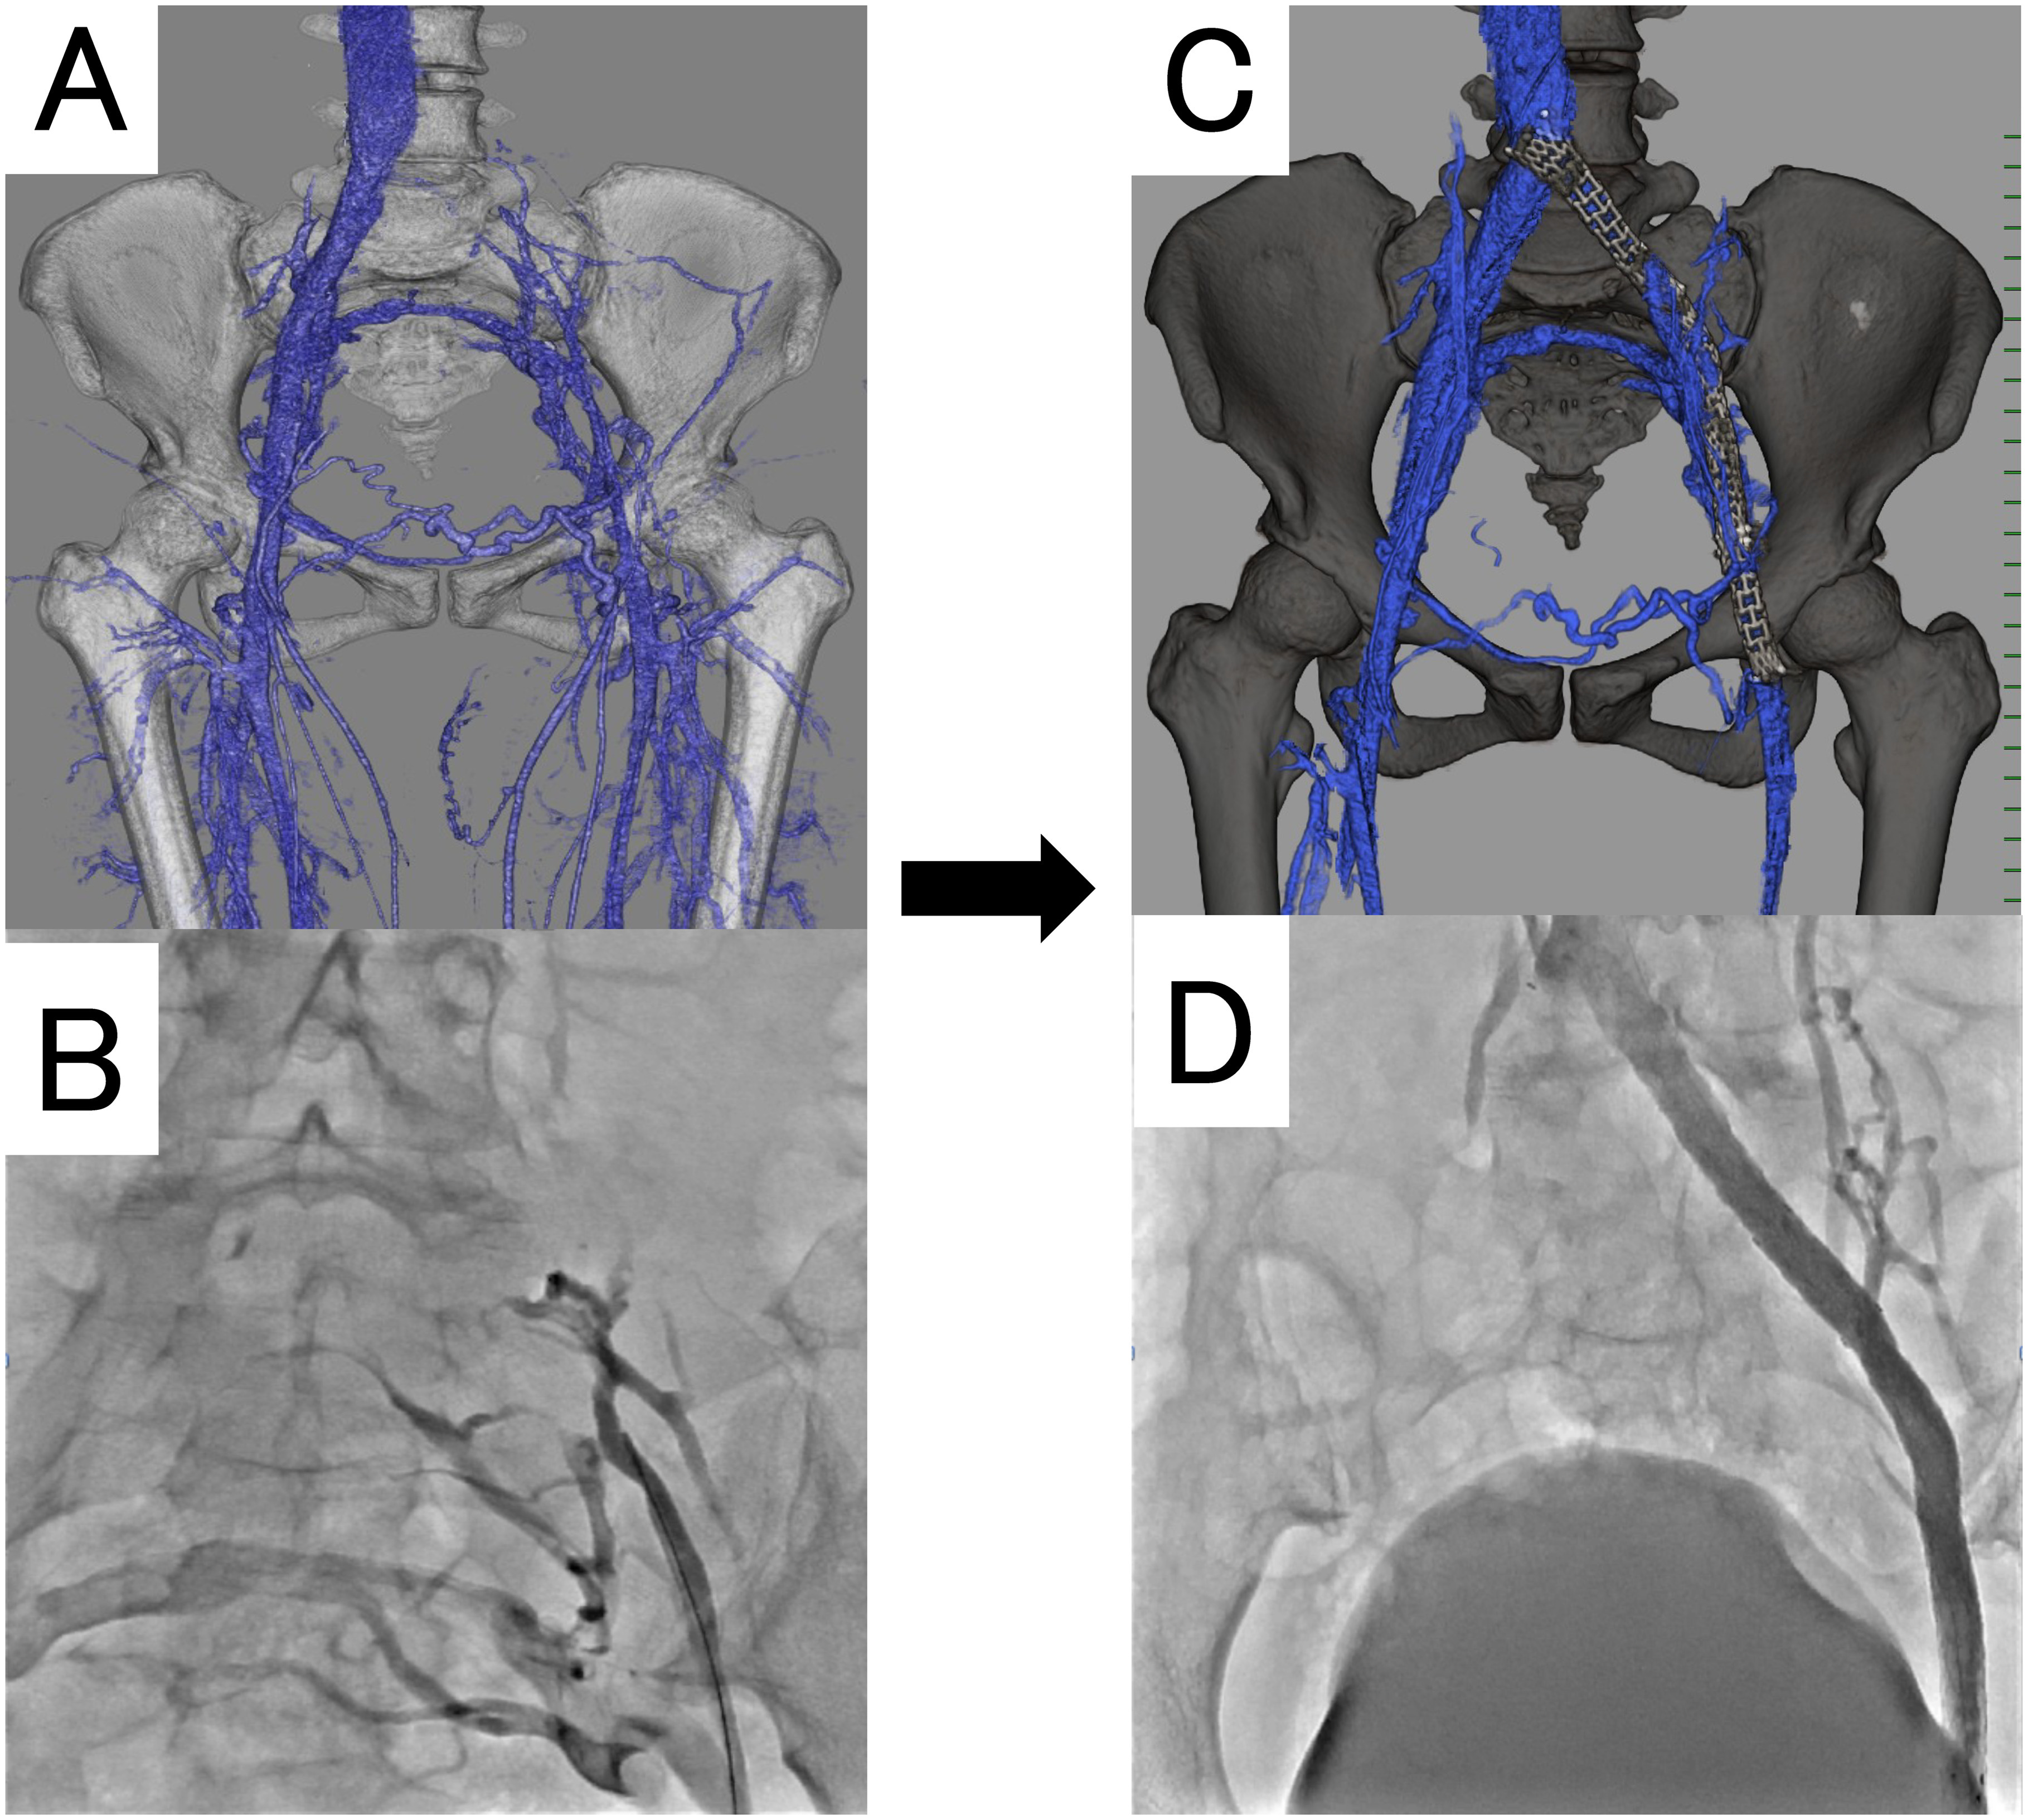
Fig. 1 Postthrombotic iliac vein occlusion and the venous stenting. (A) Contrast-enhanced computed tomography (CT) ﬁnding: before stenting. CT scan shows a typical thrombotic iliac vein occlusion with transpelvic collaterals. (B) Ascending venography ﬁndings: before stenting. Transfemoral venogram shows a typical thrombotic iliac vein occlusion with transpelvic collaterals. (C) Contrast-enhanced CT ﬁnding: after stenting. CT scan after stenting shows no stenosis or collaterals. (D) Ascending venography ﬁndings: after stenting. The stenting is carried into the left common iliac vein, external iliac vein, and common femoral vein.
